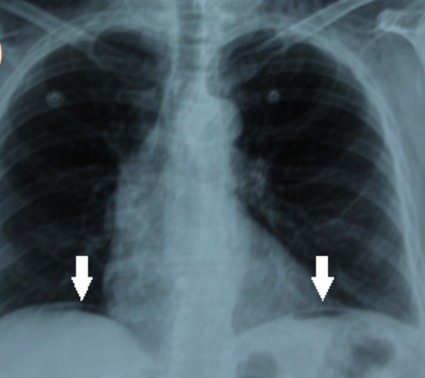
Chest X-ray at day 1 of surgical intervention. It showed significant regression of pneumoperitoneum (see arrows)
